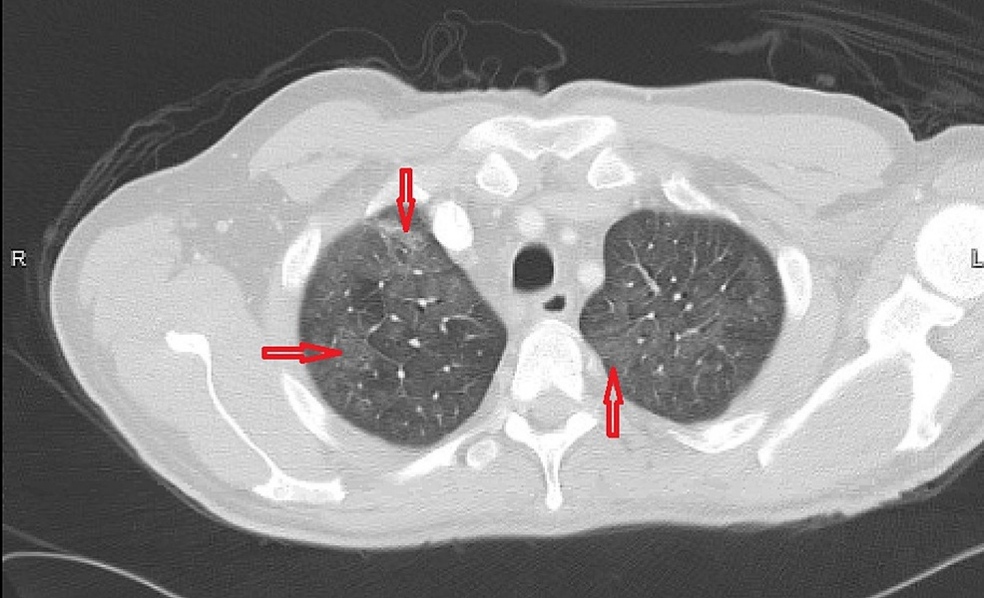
CT chest scan with IV contrast demonstrating moderate diffuse ground glass opacities (red arrows)
